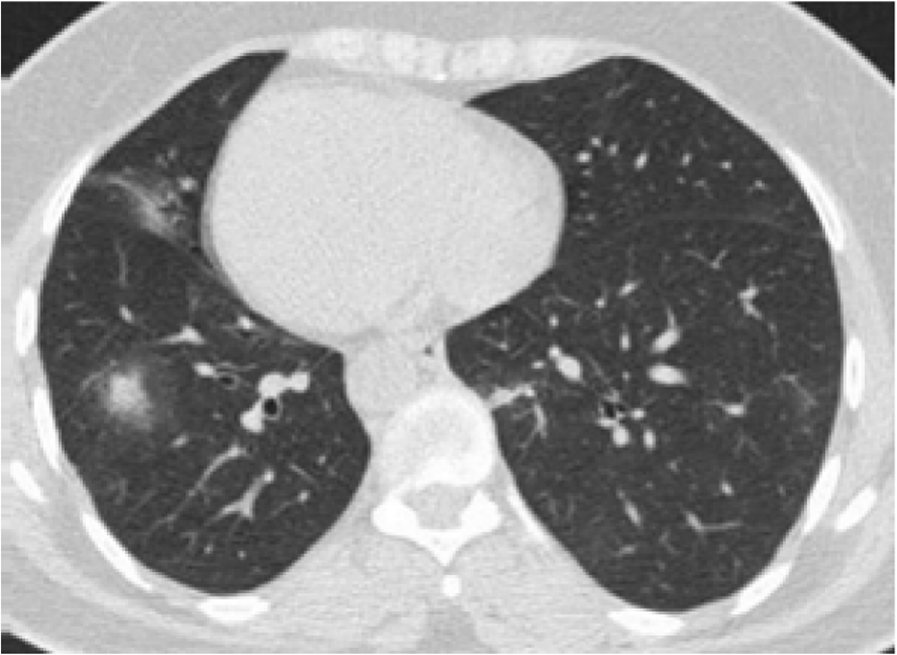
COVID-19 pneumonia: thin section CT shows nodular opacity with ground glass halo
